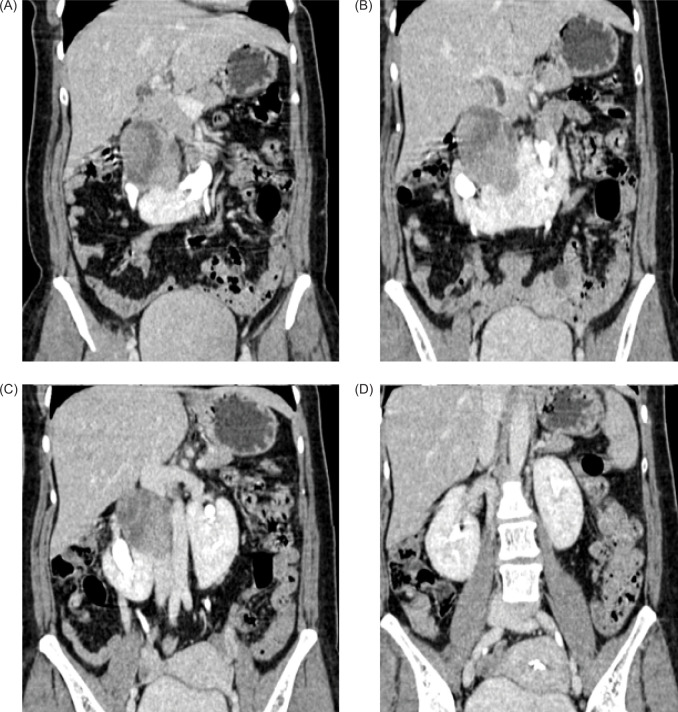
Contrast-enhanced CT coronal sections showing medially deviated lower poles of the bilateral kidneys with heterogenous enhancing mass arising from the superior portion of isthmus of the HSK abutting the hilum of right kidney.
